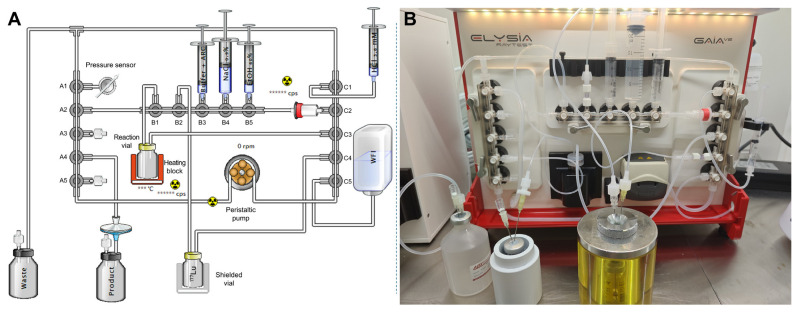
(A) Software system for the automated preparation of [^177^Lu]Lu-PSMA-ALB-56 with the GAIA^®^ synthesizer according to the optimized method; (B) Cassette setup on the GAIA^®^ module for the synthesis of [^177^Lu]Lu-PSMA-ALB-56 according to the optimized method.
